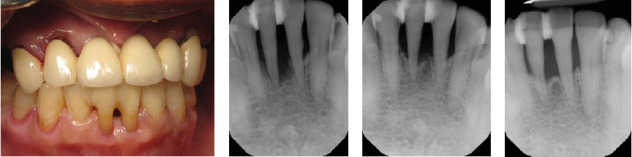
Periodontally compromised mandibular incisors were splinted in 2016 (A and B). Radiographically observed long-term stability in 2019 (C) and 2022 (D).
